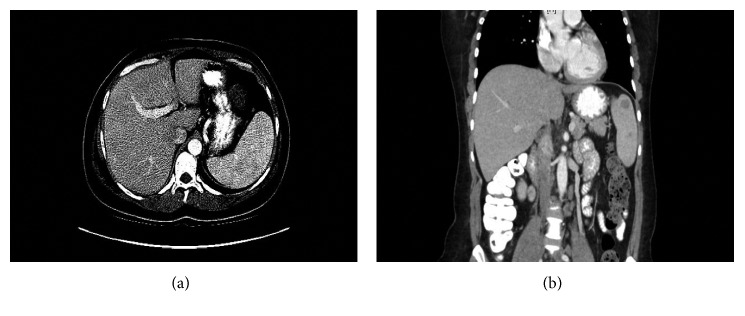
(a, b) CT scan of the abdomen showing presence of an enlarged spleen measuring 16 × 7 × 6 cm with multiple hypodense lesions.
